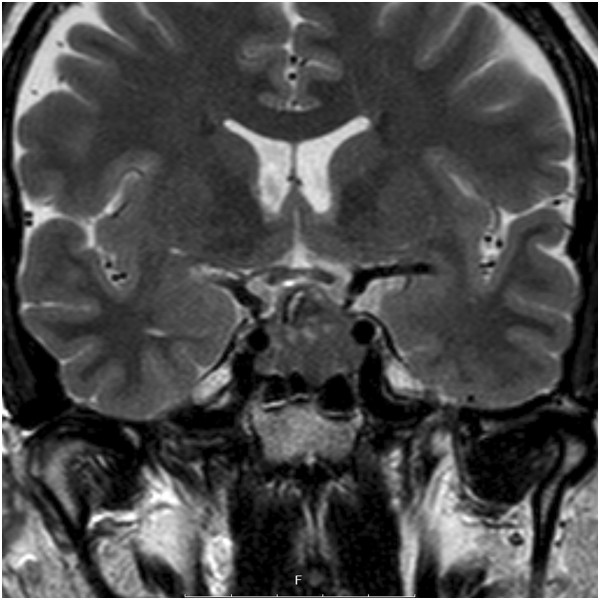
Coronal T2-weighted magnetic resonance imaging of the sella, showing a 20
